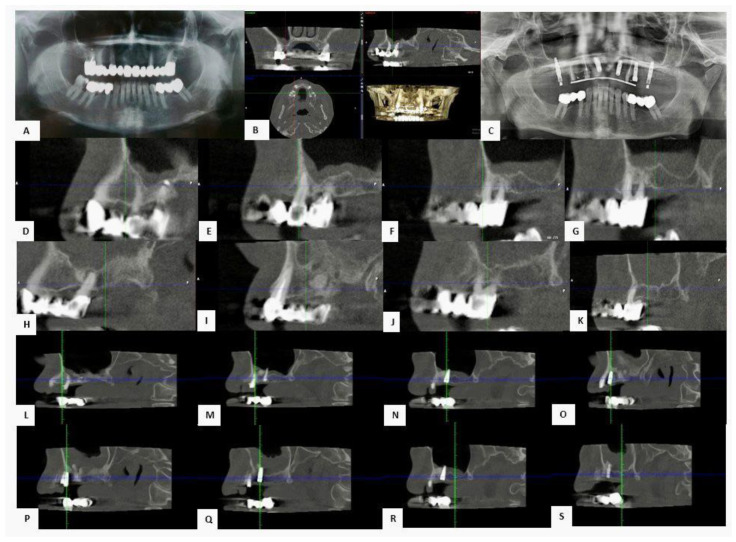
(A) Pre-operative OPT. (B) Pre-operative CBCT. (C) OPT check after 84 months. (D–K) Pre-operative CBCT sections of areas 1.4, 1.5, 1.6, 1.7, 2.4, 2.5, 2.6 and 2.7. (L–S) CBCT sections after 84 months of areas 1.4, 1.5, 1.6, 1.7, 2.4, 2.5, 2.6 and 2.7.
